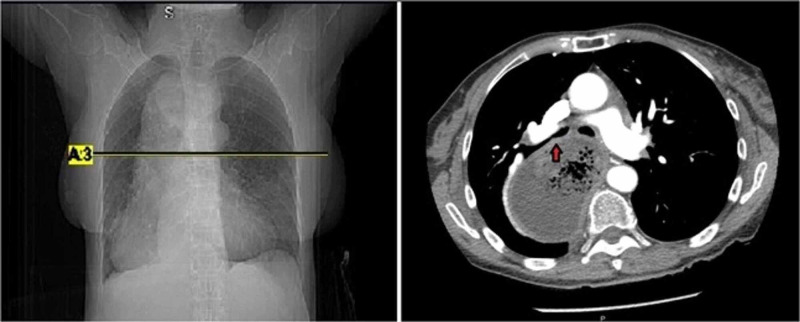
Computed tomography angiogram of the chest with an axial view (left) and corresponding coronal section (right) displaying a significantly dilated megaesophagus occupying most of the right hemithorax There is also some intraluminal fluid with a small amount of gas partially obstructing the right main bronchi (arrow).
